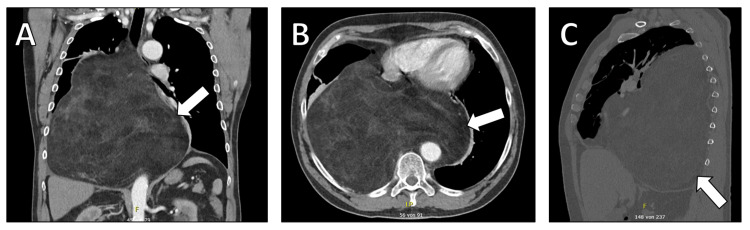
Computed tomography imaging demonstrating the liposarcoma (arrow) resected five years ago. (A) Coronal view showing the size and position of the original tumor within the thoracic cavity. (B) Transverse view highlighting the tumor's relationship to the surrounding anatomical structures. (C) Sagittal view providing a detailed perspective on the tumor's depth and its proximity to vital organs.
